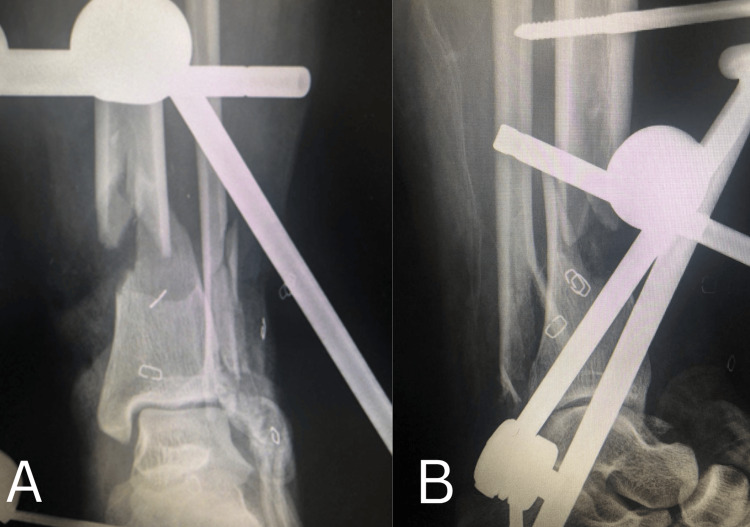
Immediate postoperative radiographs after applying an ankle-spanning external fixator A. Anteroposterior view demonstrating application of a spanning external fixator with restoration of length and alignment of the distal tibia. B. Lateral view confirming satisfactory provisional reduction and maintenance of sagittal alignment of the ankle joint.
